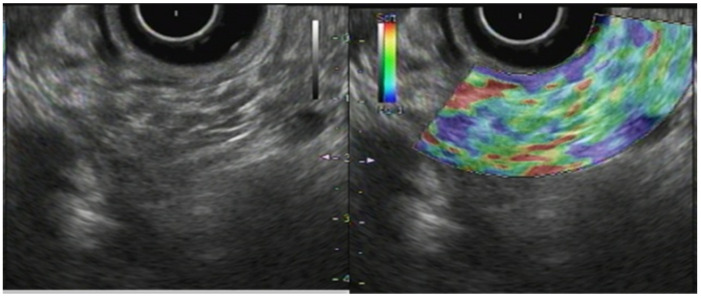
Endosonography (EUS) elastography in chronic pancreatitis.
